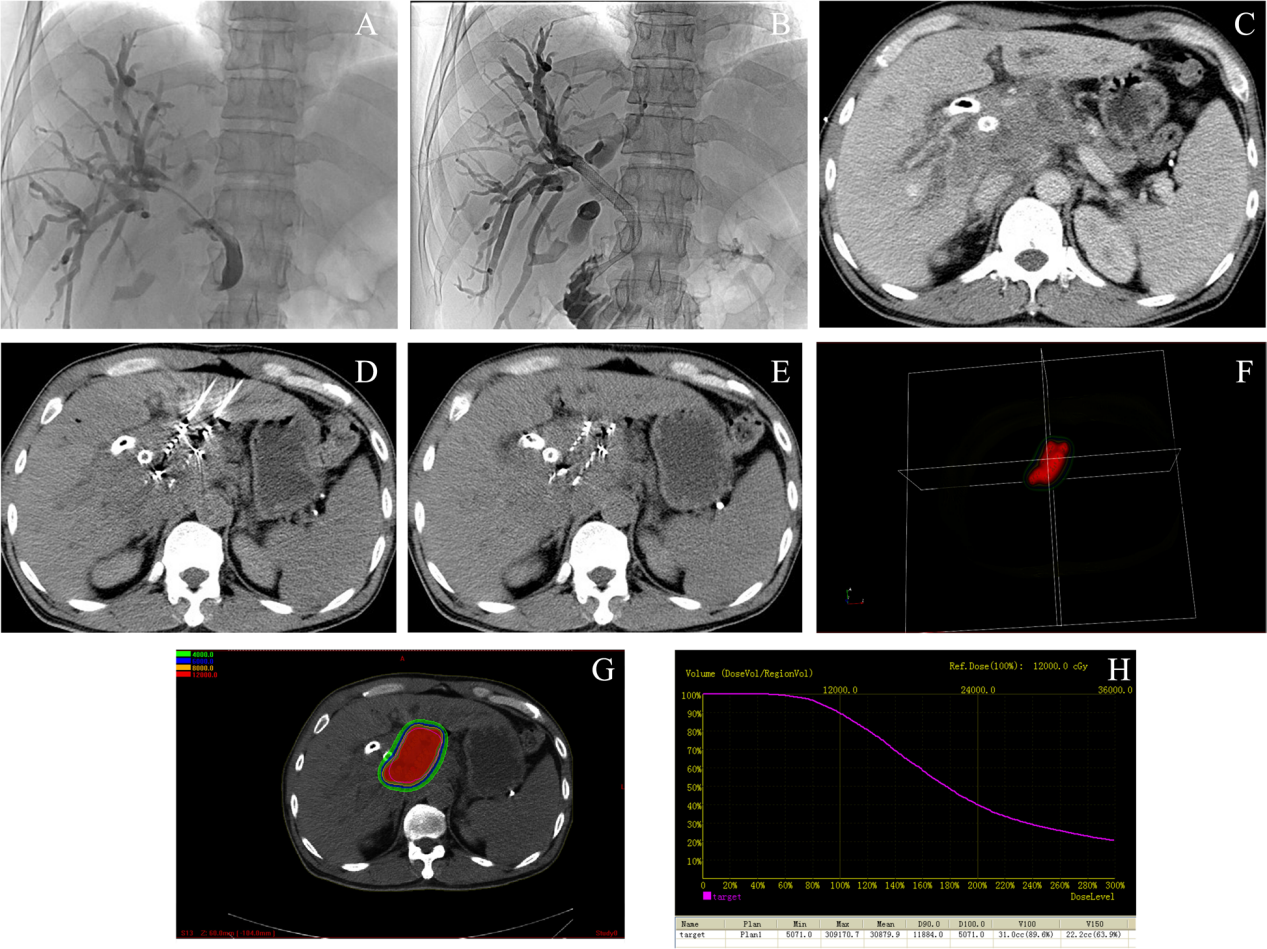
A 47-year-old male patient with cholangiocarcinoma received SEMS insertion plus CT-guided percutaneous ^125^I seed implantation. A Cholangiography showed the obstruction of the common bile duct. B Cholangiography showed an 8×80 mm self-expandable metallic biliary stent insertion. C Computed tomography showed the mass of malignancy. D Computed tomography showed the image of the percutaneous ^125^I seed implantation process. E Computed tomography showed the seeds’ distribution after ^125^I seed implantation. F Three-dimensional reconstructed image of the implanted seeds. G Dose distribution of the seeds on TPS. H Dose-volume histogram calculated after CT-guided percutaneous ^125^I seed implantation
